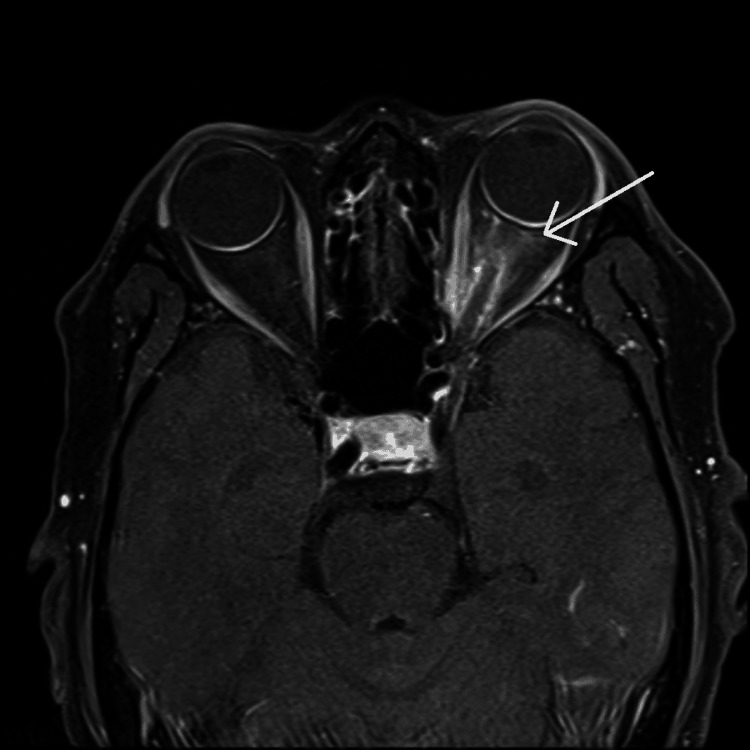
MRI of the orbits with and without contrast MRI, magnetic resonance imaging
